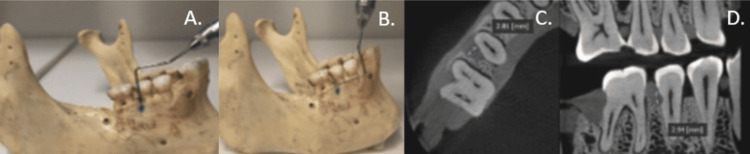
Measurement of two-wall defect. A. Superior-inferior measurement (dry mandible), B. Mesiodistal measurement (dry mandible), C. Mesiodistal measurement (cone beam computed tomography (CBCT)), D. Superior-inferior measurement (CBCT)
